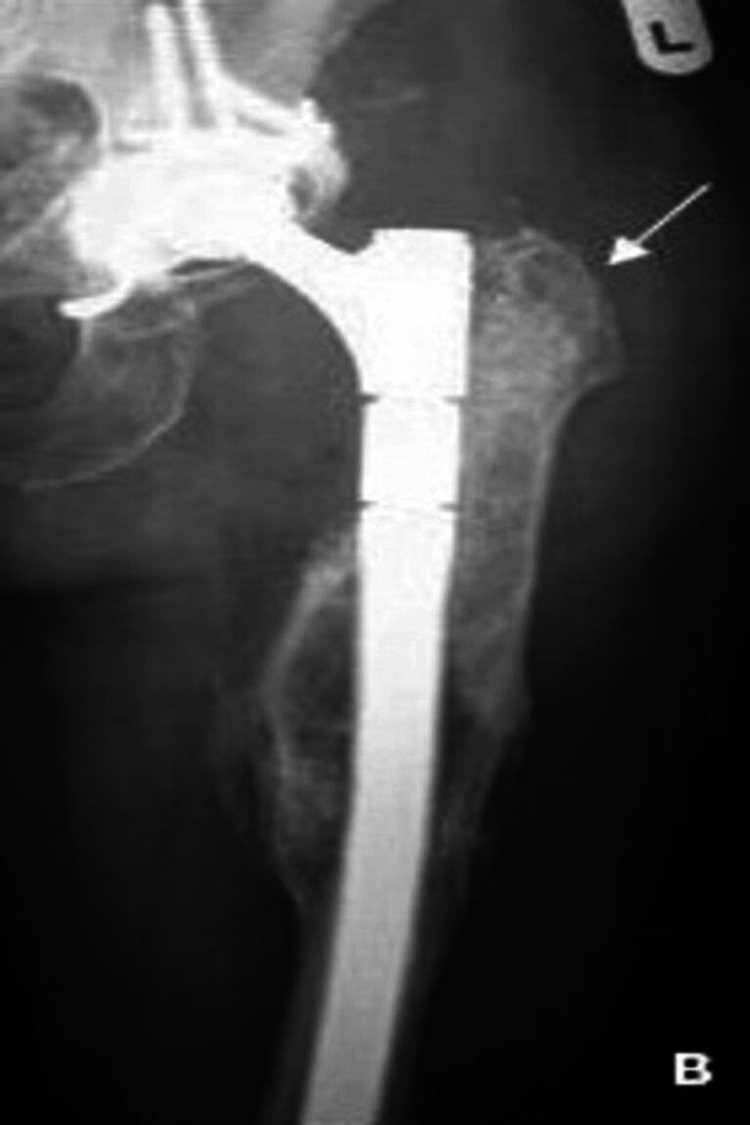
Uncemented stem Follow-up radiograph two years after a modular revision prosthesis titan uncemented stem revision with femoral impaction grafting and distal diaphyseal fixation. This image is reproduced from Wimmer MD, Randau TM, Deml MC, Ascherl R, Nöth U, Forst R, Gravius S. 2013; 14 [3].
